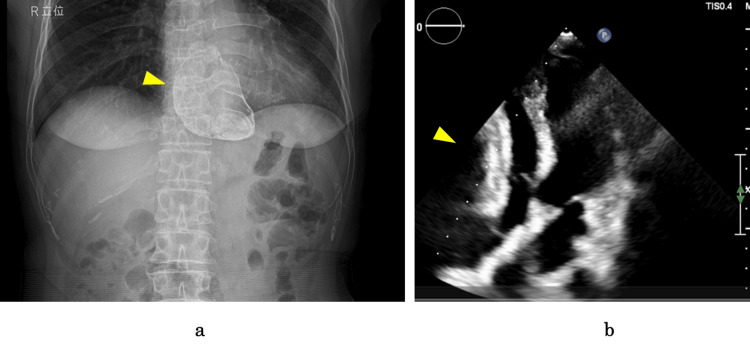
Preoperative X-ray image and echocardiography (a) X-ray image revealing a calcified mass within the cardiac shadow (arrow). (b) Transthoracic echocardiography demonstrating a mass anterior to the heart (arrow), resulting in right ventricular compression and diastolic dysfunction. This figure shows an apical four-chamber view.
